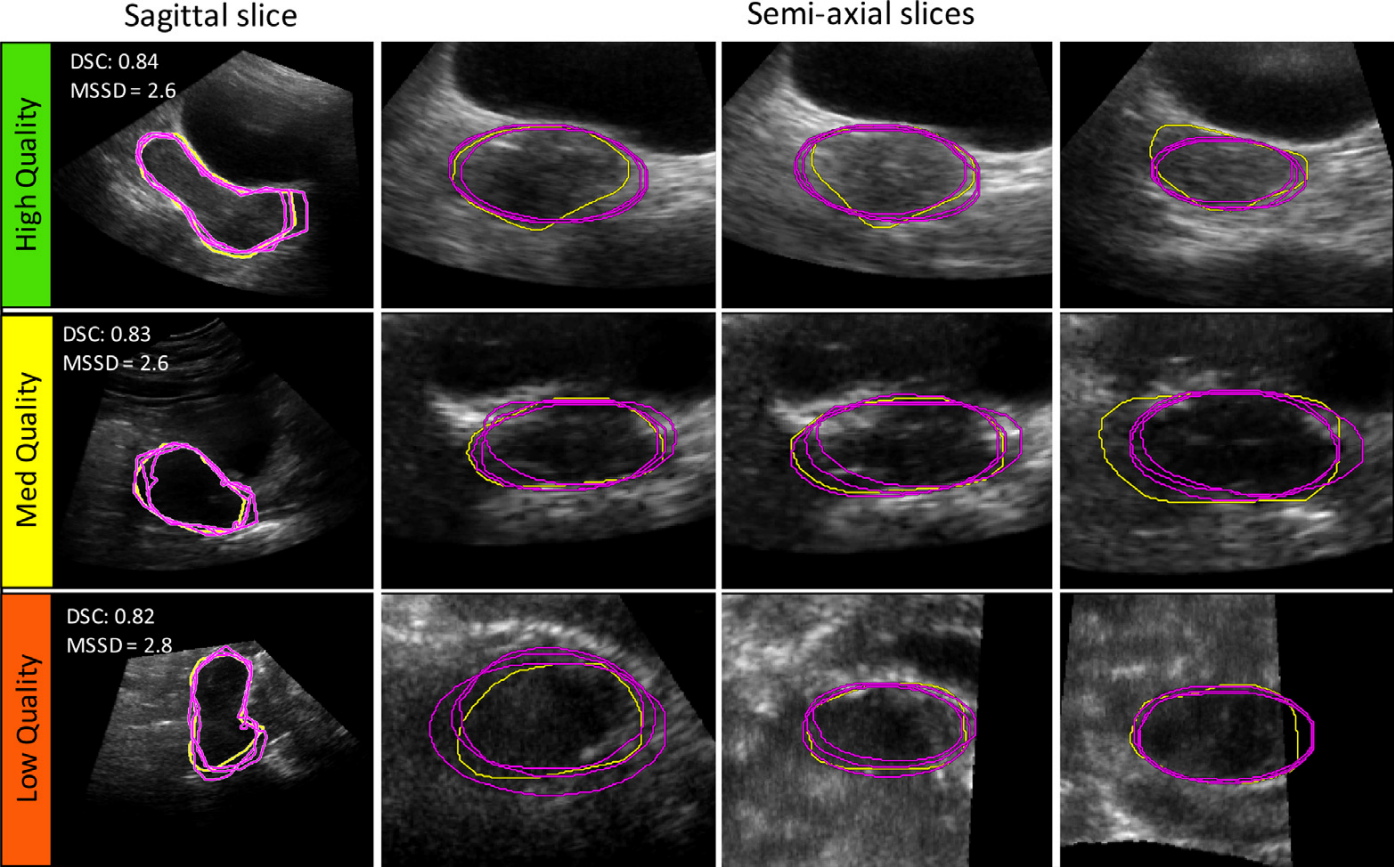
Example segmentations using the stacked-ellipse (SE) algorithm (*magenta,* three observers) compared with the corresponding gold standard manual segmentation (*yellow*). Each row contains example 2-D cross sections from the final 3-D segmentation in various orientations for high, medium and low ultrasound image quality. The Dice similarity coefficient (DSC) and mean absolute surface-to-surface distance (MSSD, mm) for each segmentation are displayed on the sagittal slice.
